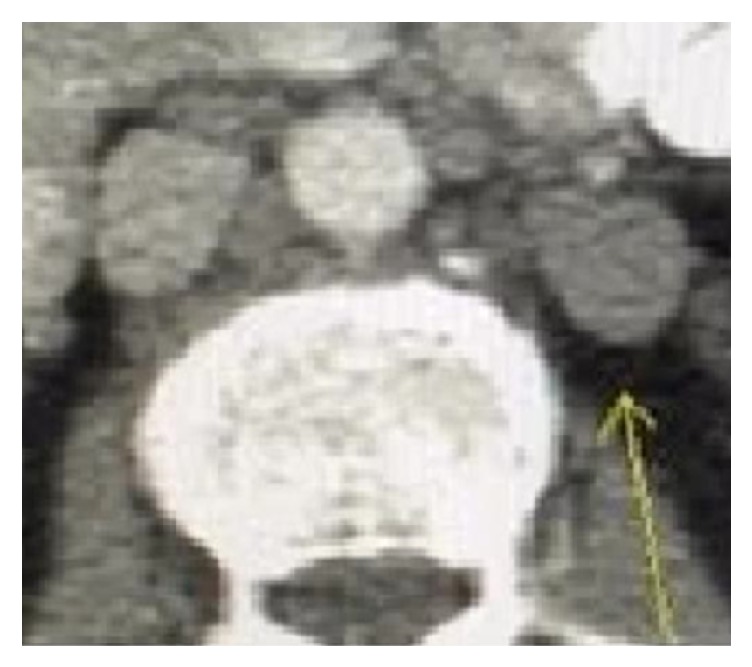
CT scan revealing para-aortic lymphadenopathy.
